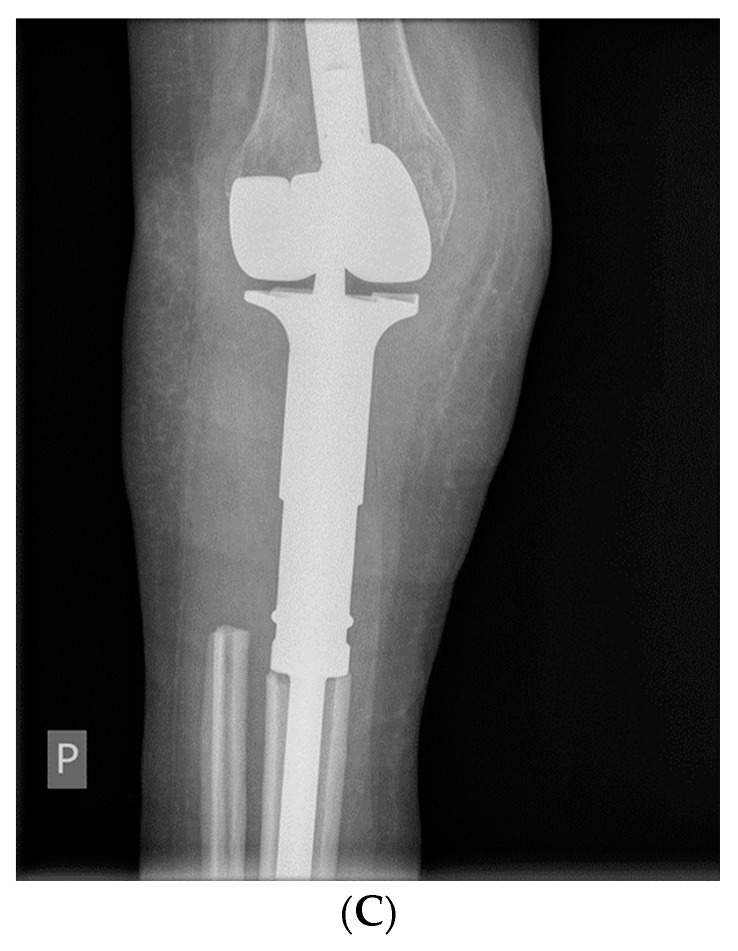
Significant calcification of the locally advanced GCTB of proximal tibia after 8 doses of denosumab (X-rays). Prolonged denosumab treatment can aid resection of the initially inoperable cases. (A): Lesion before denosumab therapy, (B): after 8 doses of denosumab, (C): after the surgery with radical resection and implantation of oncological prosthesis.
